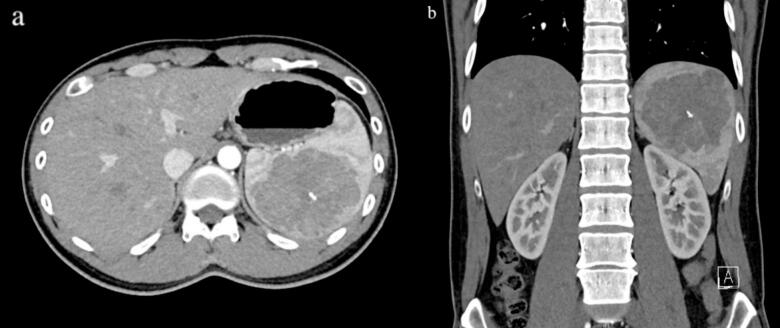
Abdominal CT: large splenic mass with intralesional calcification, r/o SANT (a) axial, (b) coronal view.
